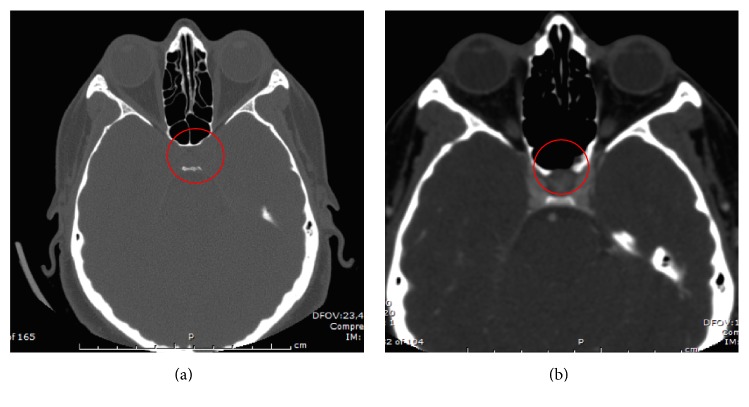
Case 4. (a) Preoperative CT scan showing a pituitary adenoma. (b) Seven-month postoperative CT showing a doubtful image of bony closure of the surgical defect.
